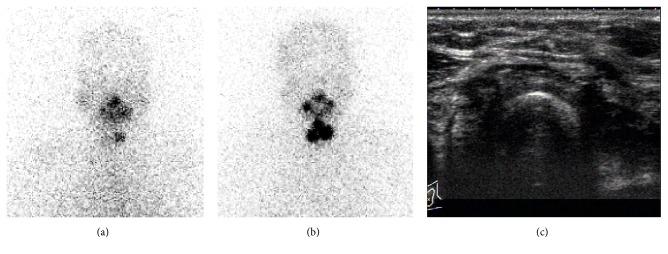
Comparing diagnostic ^131^I scan with postablation therapy ^131^I imaging. A 49-year-old female after total thyroidectomy. (a) Diagnostic RAI scan performed 24 hours after 74 MBq (2 mCi) ^131^I oral administration. (b) Therapeutic dose scan performed 3 days after 3.7 GBq (100 mCi) ^131^I oral administration 3 weeks after diagnostic ^131^I scan. Thyroid remnant is visualized by diagnostic and therapeutic dose of ^131^I scans, but smaller residual thyroid is found by diagnostic scan. (c) Ultrasound fails to detect thyroid remnant.
